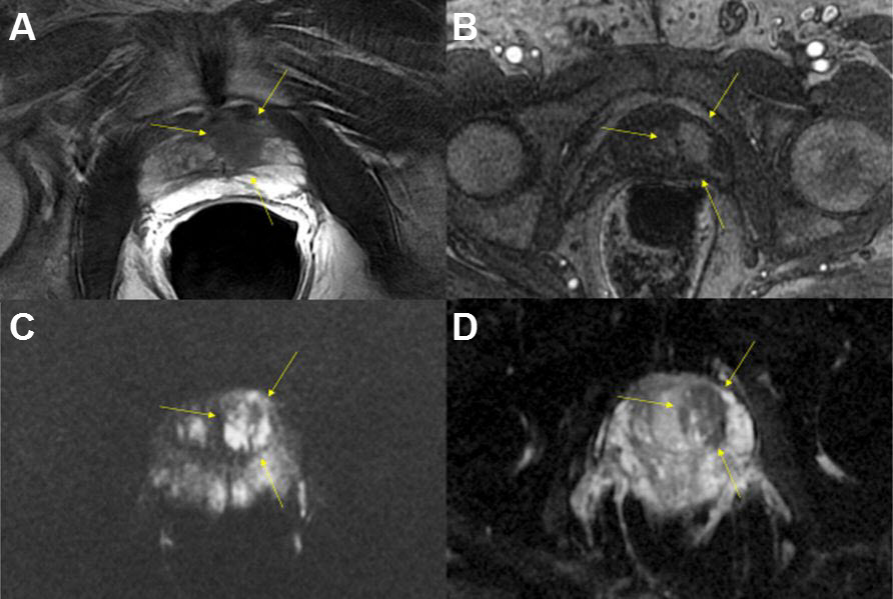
Multiparametric MRI of the Prostate of a 65-Year-Old Man Shown are a transitional zone lesion with low signal on T2-weighted images (A), early avid enhancement on T1-weighted images after gadolinium injection (B), high signal on b=1400 ms diffusion-weighted images (C), and low signal on ADC map (D). Detection of lesions in this location is challenging with transrectal ultrasound, but feasible with mpMR.
